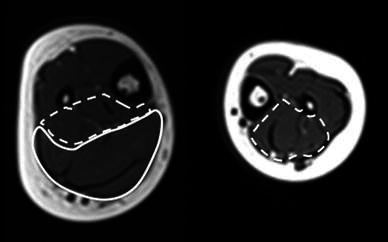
Magnetic resonance imaging scan of both limbs showing absent musculature in the left. Axial T1-weighted spin-echo (TE-11 TR-656) sequences through the proximal aspect of both lower limbs demonstrating the absent superficial posterior compartment on the *left*. Note the compensatory hypertrophy of the deep compartment and peroneal compartment muscles on the *left*. The *solid line* represents the superficial posterior compartment. The *interrupted line* represents the deep posterior compartment
